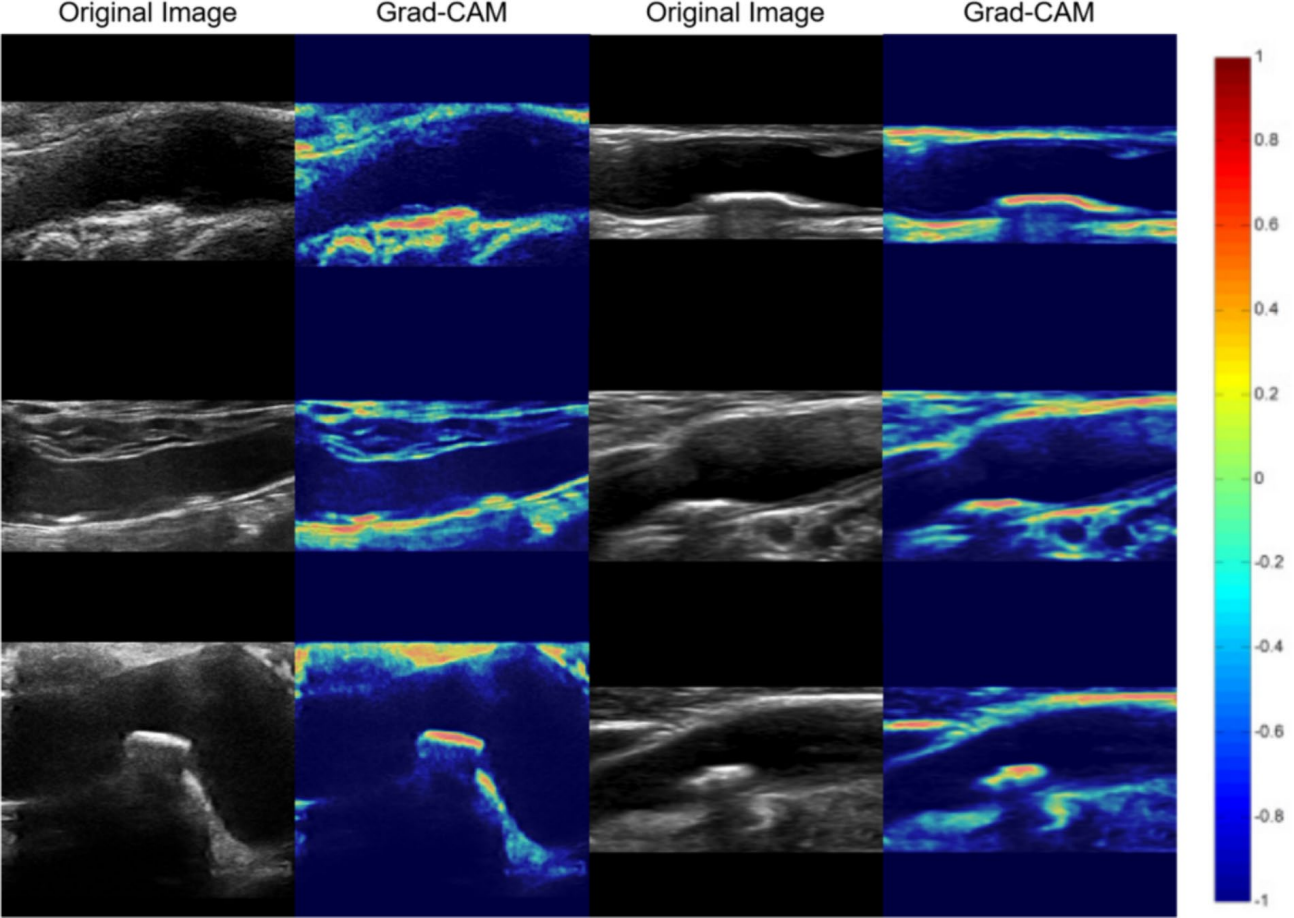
Gradient-weighted class activation mapping to identify areas of ultrasound images that the BCNN-ResNet algorithm weighed more during its calculations. Original ultrasound images that served as input are shown in grayscale, while the neighboring image is colored according to where the algorithm “focused.”
